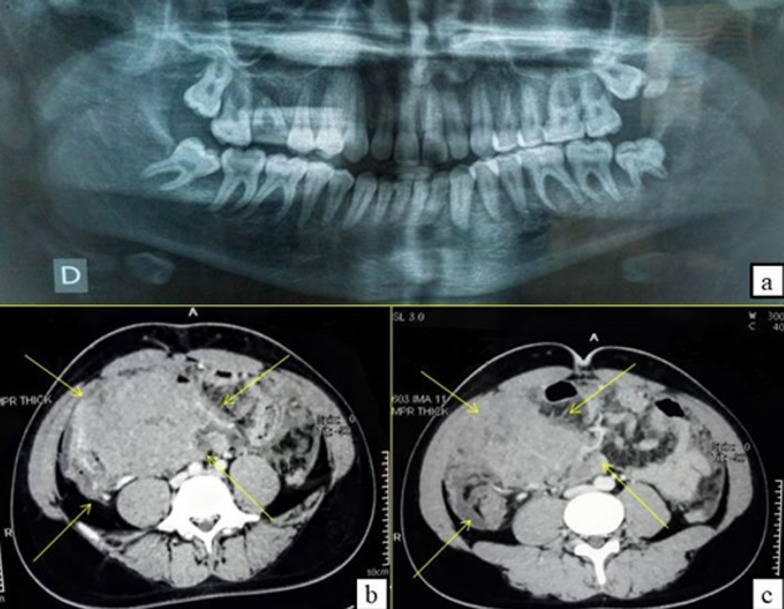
panoramic X-ray invasion of the teeth furcation and bone lysis in the left molar region (B) and abdominal CT-scan (B,C): axial sections, narrow window: tumour mass of the ileocecal junction associated with peritoneal carcinomatosis, presence of multiple mediastinal lymphadenopathies
